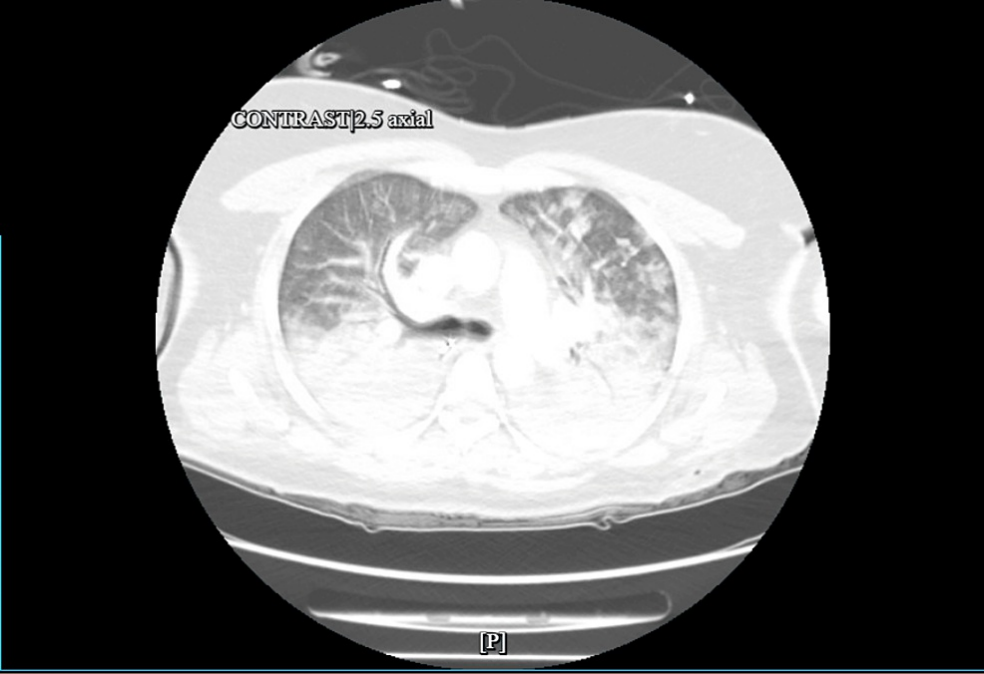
CT Scan on admission demonstrating bilateral ground glass opacities and air bronchograms
